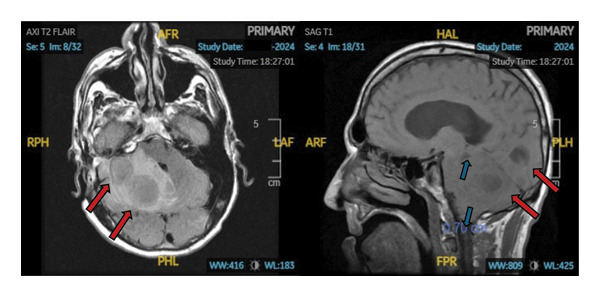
MRI of the brain on admission. Images show two peripherally enhancing masses in the right cerebellar hemispheres, with the lateral lesion measuring up to 2.6 cm and the medial lesion measuring up to 3.3 cm (red arrows). There is also significant mass effect with downward (cerebellar tonsils lie 7 mm below the foramen magnum on the right and 6 mm on the left) and upward herniation, effacement of the cerebral aqueduct, and acute noncommunicating hydrocephalus (blue arrows).
